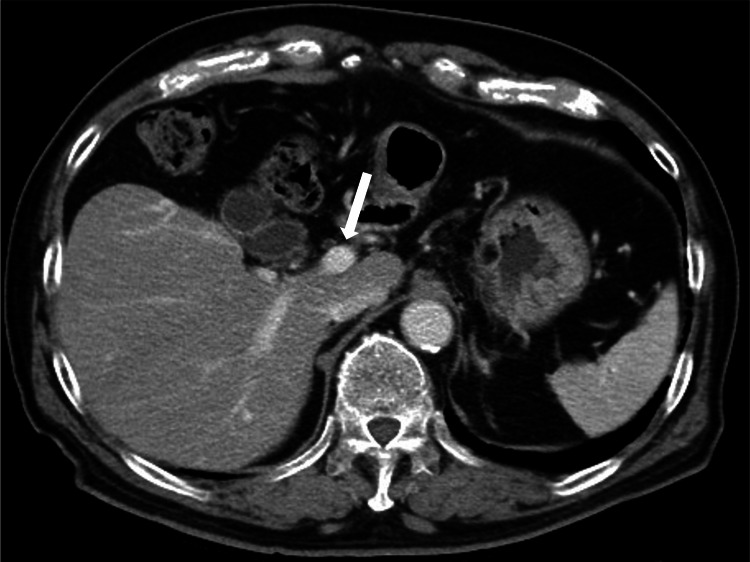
Contrast-enhanced CT imaging after 6 months Portal vein thrombosis disappeared after 6 months of treatment with rivaroxaban (white arrow) CT: computed tomography
